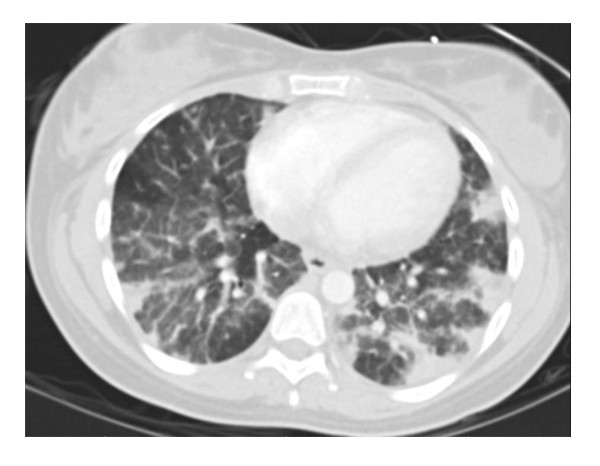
Computed tomography scan of the chest performed on hospital day three revealing bilateral, peripheral reticulonodular opacities consistent with septic pulmonary emboli.
